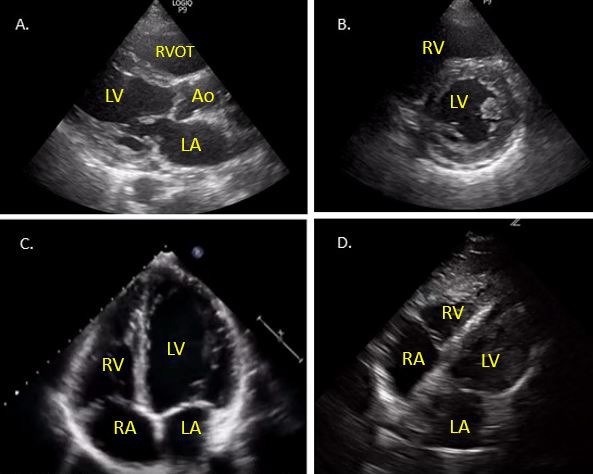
A) Parasternal long axis view. B) Parasternal short axis view. C) Apical 4-chamber view. D) Subcostal 4-chamber view. LA - left atrium, LV - left ventricle, RA - right atrium, RV - right ventricle, RVOT - right ventricular outflow tract,Ao - aorta.
